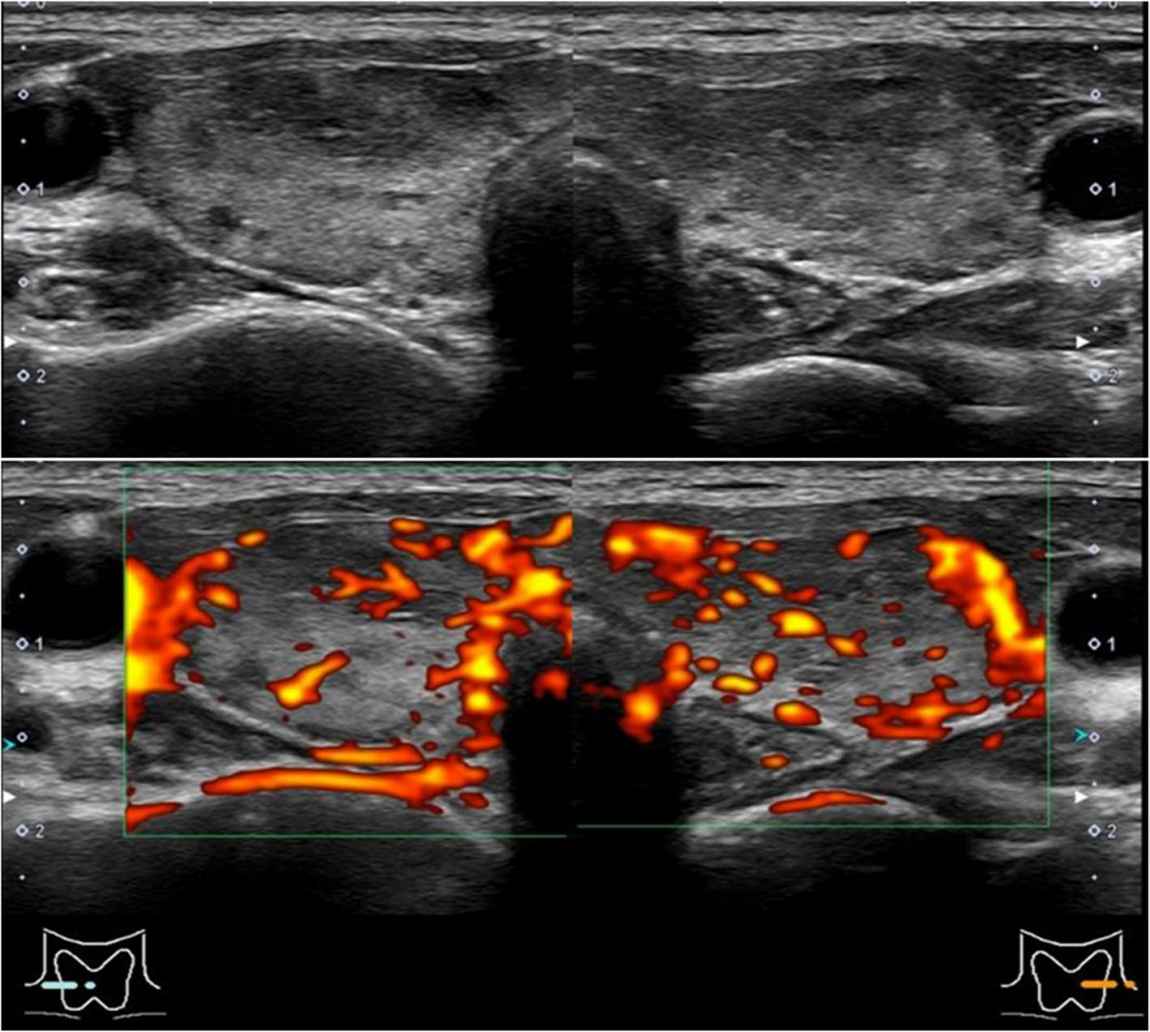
Thyroid ultrasonography. Right lobe: 15 × 50 × 13 mm. Left lobe: 13 × 50 × 11 mm. Increased blood flow throughout the thyroid gland without diffuse goiter was revealed
